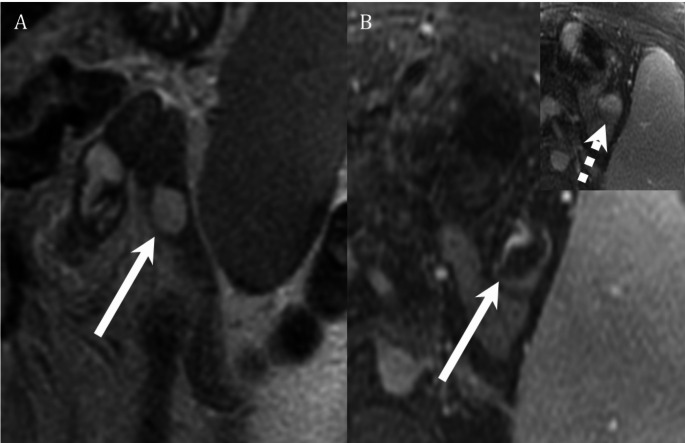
70-year-old female being evaluated with abdominal MRI for cirrhosis. A Coronal T2 SSFSE demonstrates a mass within the jejunum with high T2 signal, similar to that of luminal fluid (arrow, A). B Axial post gadolinium T1FS images in the portal venous and delayed phases (inset image, B) demonstrate slow, progressive peripheral enhancement (white arrow, B) with complete fill in on the delayed phase (dotted arrow, inset image). The finding was suspected to represent a hemangioma or slow flow vascular malformation and was unchanged on 1 year follow up MRI (not shown)
